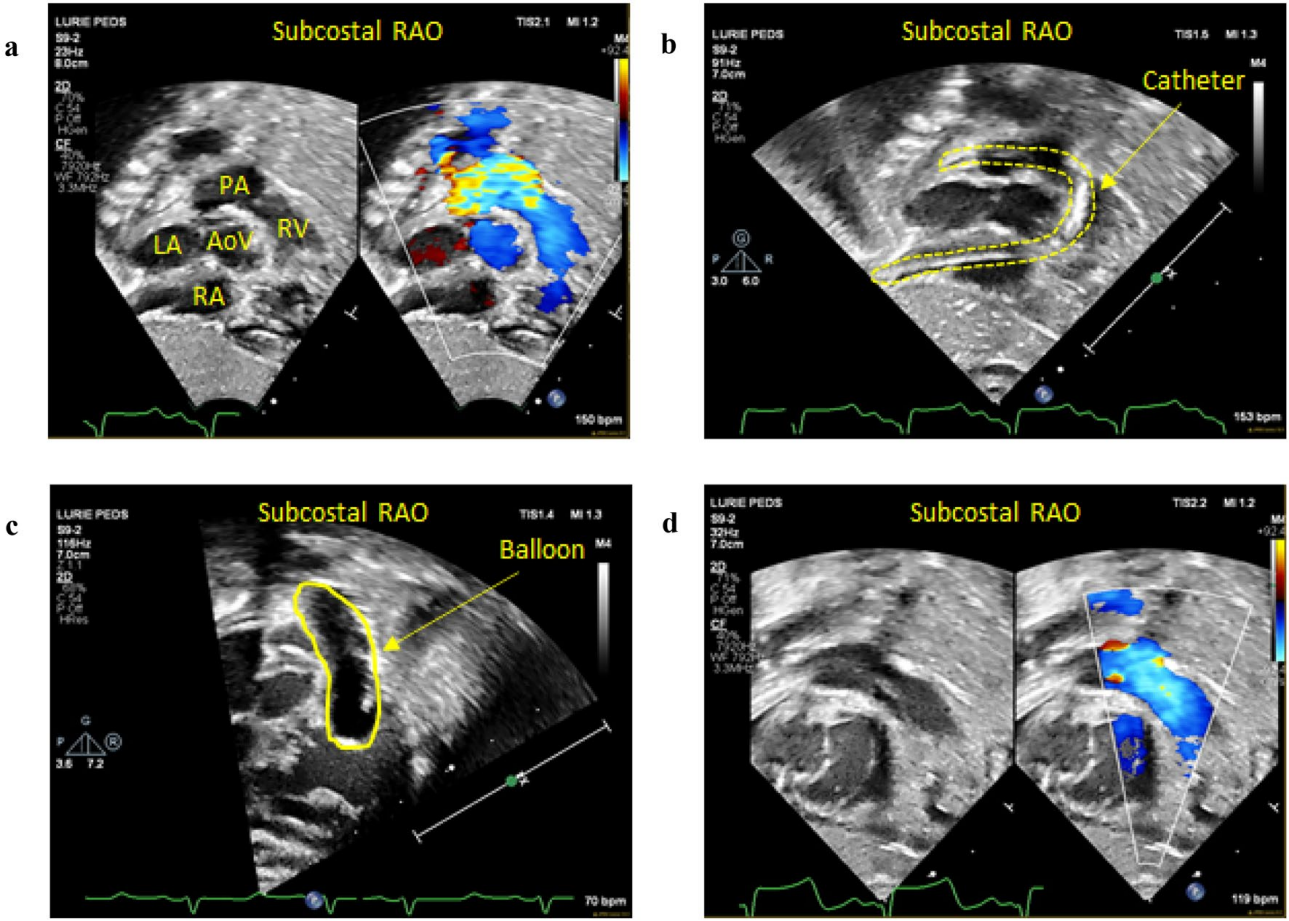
Key intra-procedure echocardiographic findings during EG-PBPV. a Baseline assessment showing increased flow velocity by color Doppler starting at the level of the pulmonary valve without sub- or supravalvular pulmonary stenosis. b Subcostal RAO view showing catheter coursing from IVC across TV through RVOT and into RPA (highlighted with dashed lines). c Subcostal RAO view showing balloon inflated across pulmonary valve (outlined in yellow). Balloon is not impinging on TV. d Following balloon valvuloplasty, the flow acceleration across the pulmonary valve has resolved. *RAO* right anterior oblique, *RA* right atrium, *RV* right ventricle, *PA* pulmonary artery, *LA* left atrium, *AoV* aortic valve
